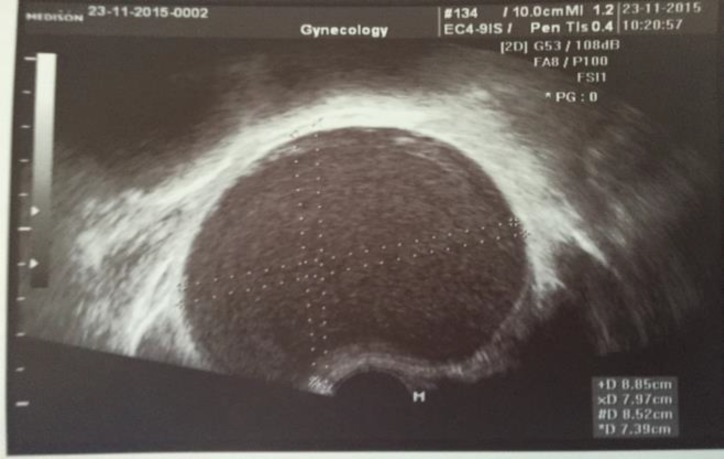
Transvaginal ultrasound, view of the suspicious cyst
